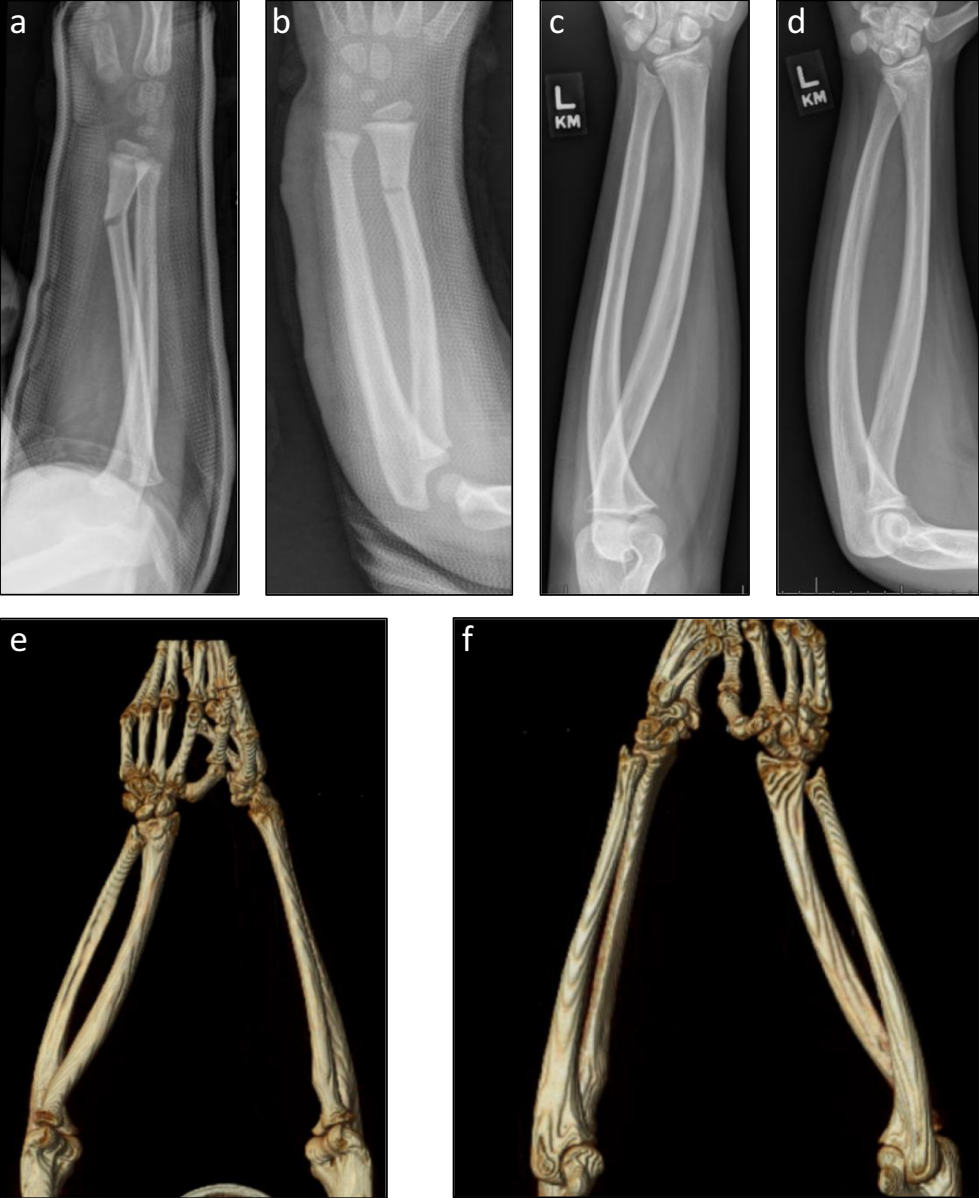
Preoperative imaging of patient in Fig. 2. a–b Orthogonal radiographs of left forearm after closed reduction and long arm casting at 3 years of age. c–d Radiographs of affected forearm at 15 years of age. e Three-dimensional rendering of CT of both forearms viewed from cephalad to caudad. f Same rendering viewed from caudad to cephalad. (Courtesy of Sanjeev Sabharwal, MD, MPH)
